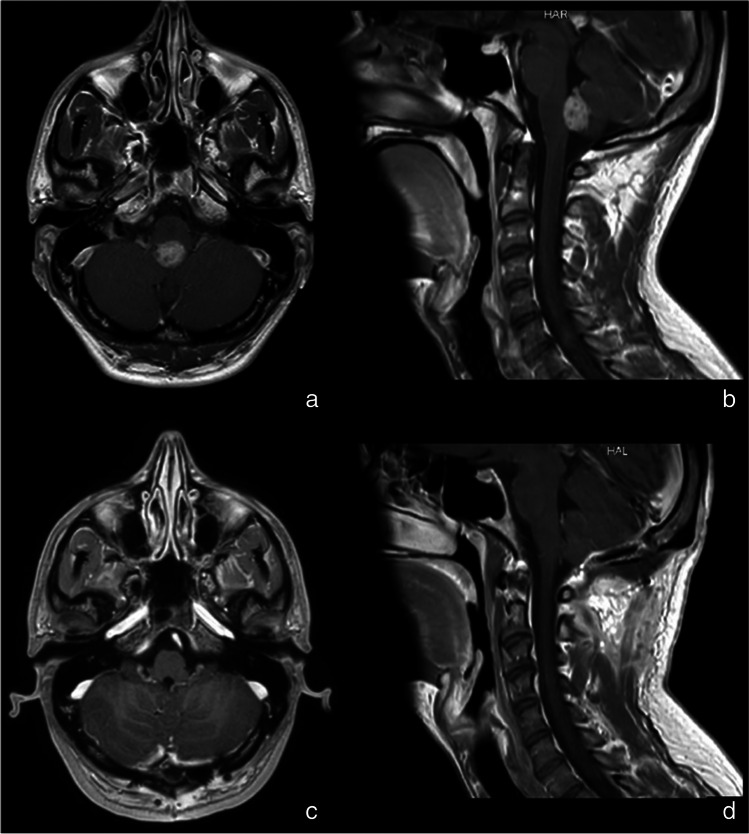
Preoperative (a and b) and postoperative (c and d) images of patient number 3 who underwent 5-ALA-guided surgery. T1-weighted image with gadolinium shows complete resection of the lesion
